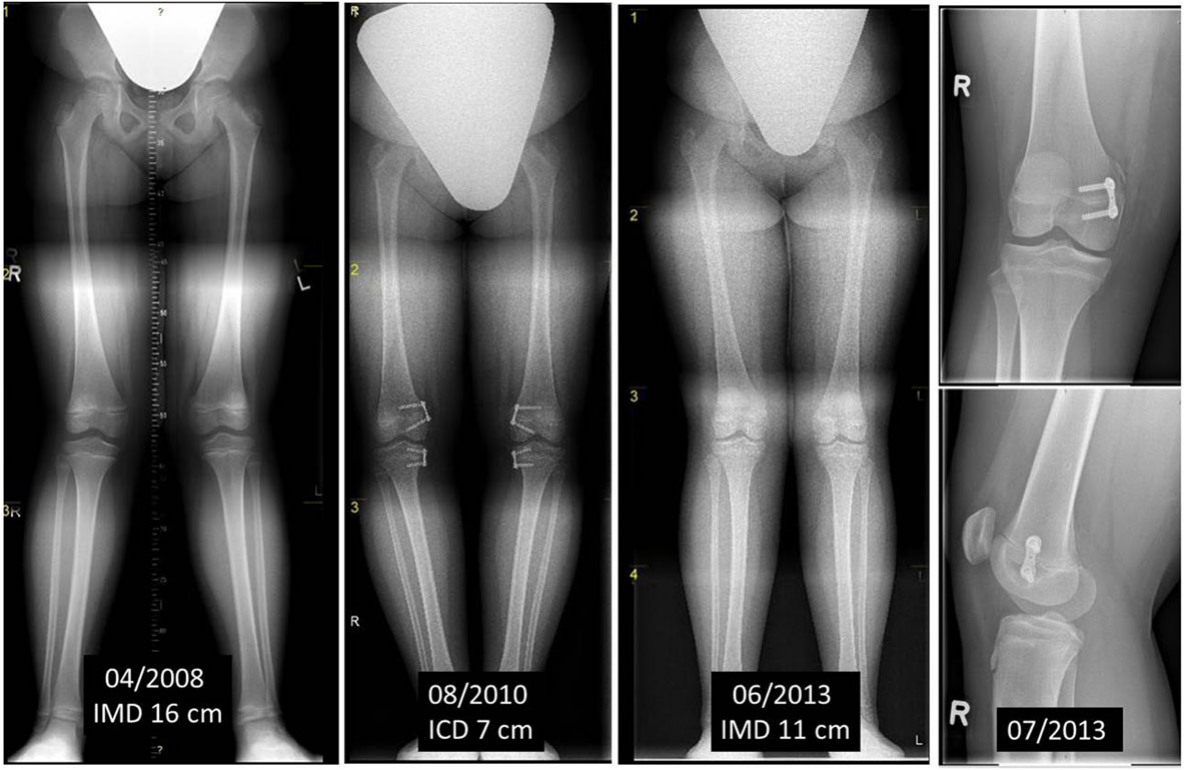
9-year-old adipose girl with genu valgum (IMD 16 cm) treated with femorotibial eight-Plates in April 2008 and growth enabled following material removal upon overcorrection (ICD 7 cm) in August 2010. Clinical relapse with IMD 11 cm in June 2013 with femoral error determined by X-ray and repeated hemiepiphysiodesis
